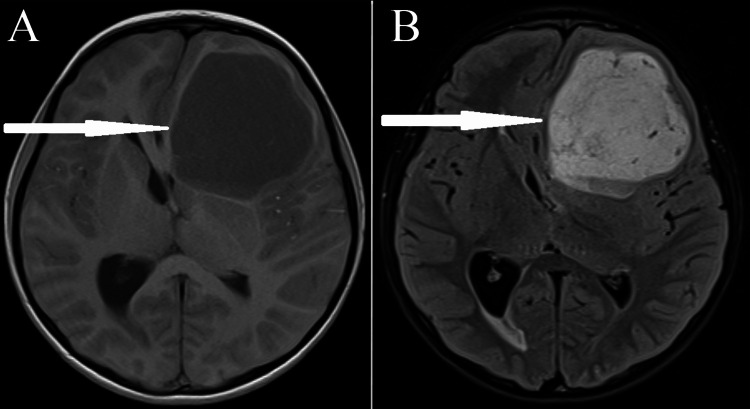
Images from the MRI scan: glioblastoma located in the left frontal lobe (A) T1 sequence and (B) T2 sequence
